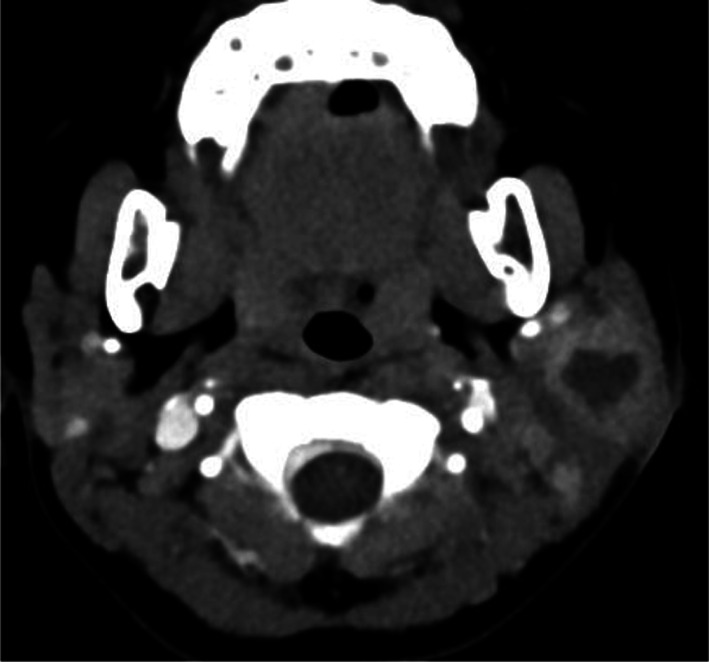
Left parotid gland abscess on CT, after intravenous injection of contrast medium
